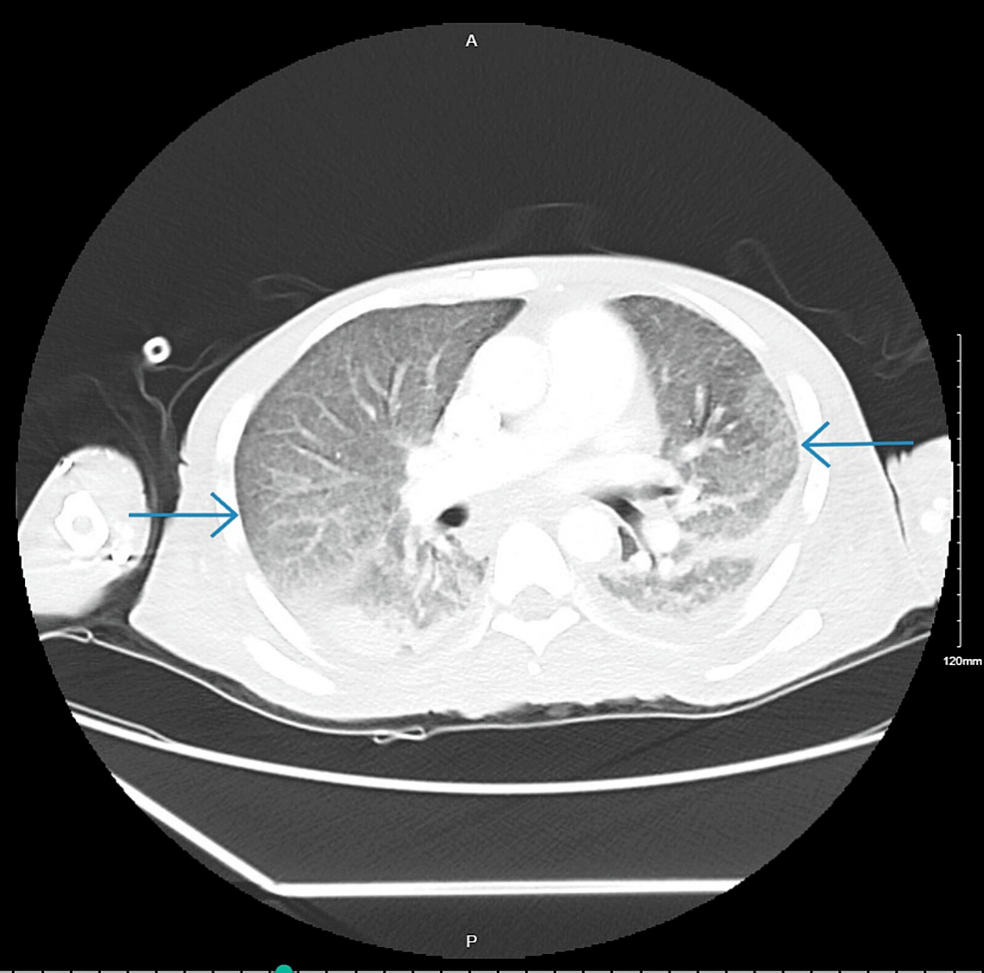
CT chest with contrast showing diffuse bilateral ground-glass opacities (blue arrows).
